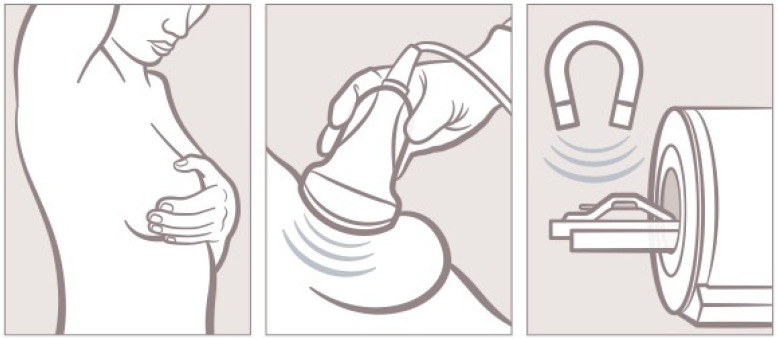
Pictography of possible tools for regular implant control. Self-examinations are easily performed frequently. Ultrasound examinations of the breast are to be conducted at least annually. MRI examination can be performed additively or carried out directly for implant follow-up.
